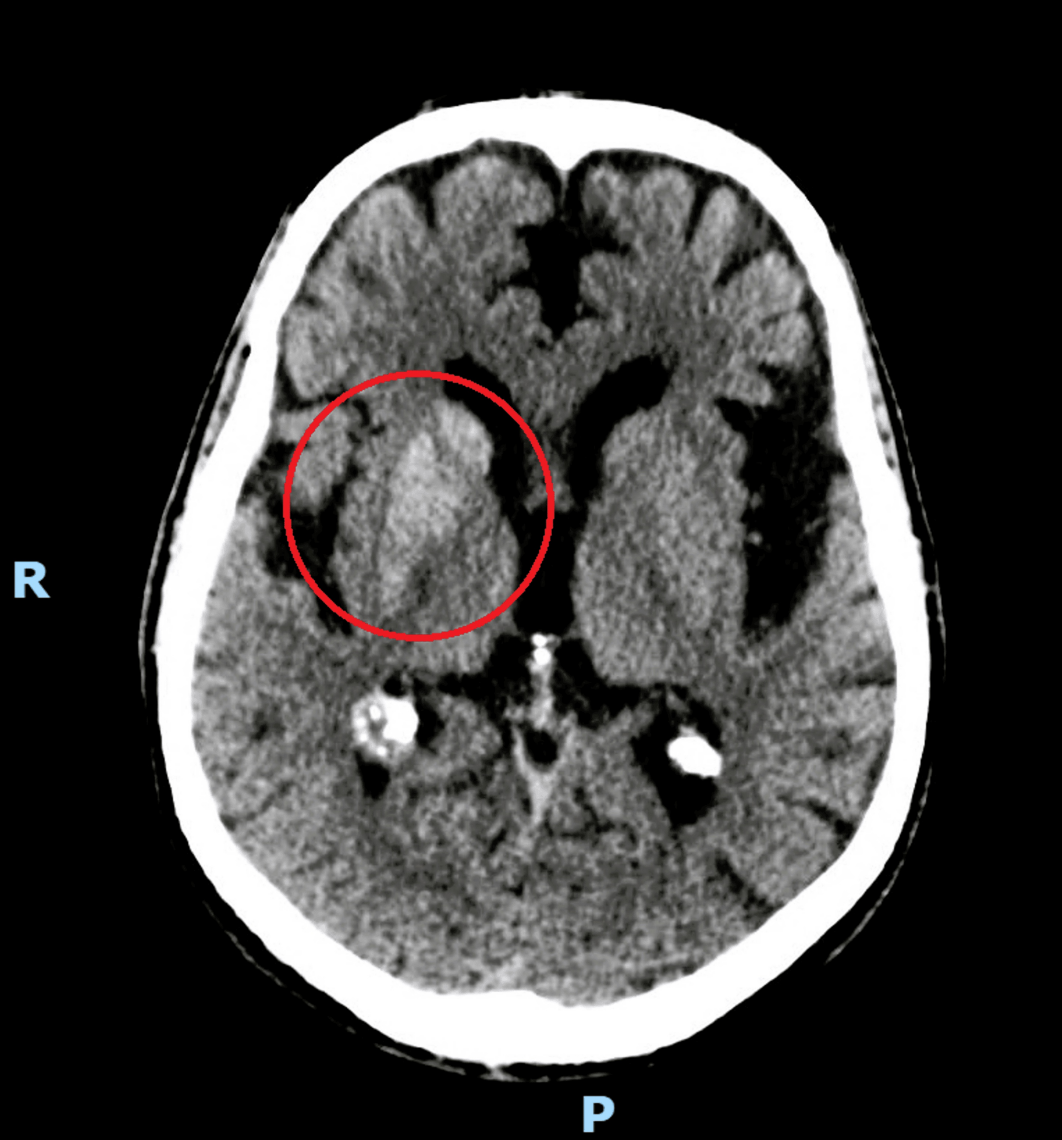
Single axial non-contrast head CT shows a discrete hyperdensity involving the right caudate and lentiform nucleus, circled in red This non-contrast head CT scan was performed with 3 mm slices parallel to the orbitomeatal plane.
